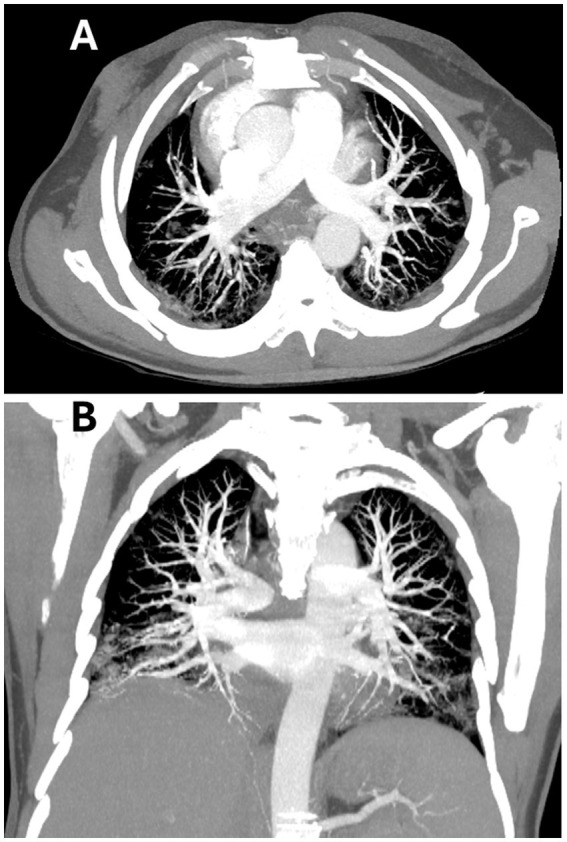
Axial (A) and coronal (B) computed tomography pulmonary angiogram images demonstrating bilateral peripheral pulmonary emboli with partial luminal occlusion. The posterior descending branches of both pulmonary arteries are seen partially obliterated by thrombotic material with normal contrast opacification of the distal segments denoting partial occlusion, with moderate pericardial effusion, and regressing bilateral interstitial COVID-19 pneumonia.
